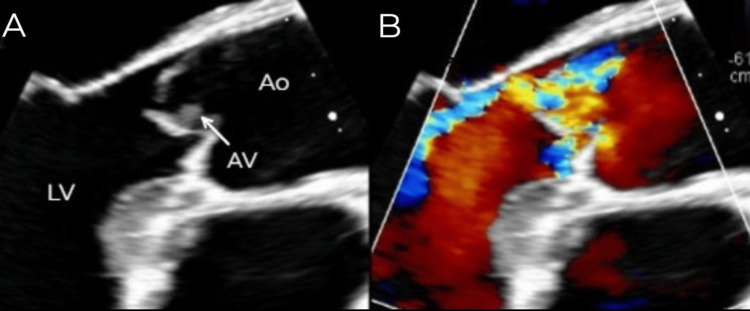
Transoesophageal echocardiogram. (A) Structural view demonstrating the aorta (Ao), aortic valve (AV), and left ventricle (LV). (B) Colour Doppler view demonstrating a broad regurgitant jet consistent with severe aortic regurgitation.
